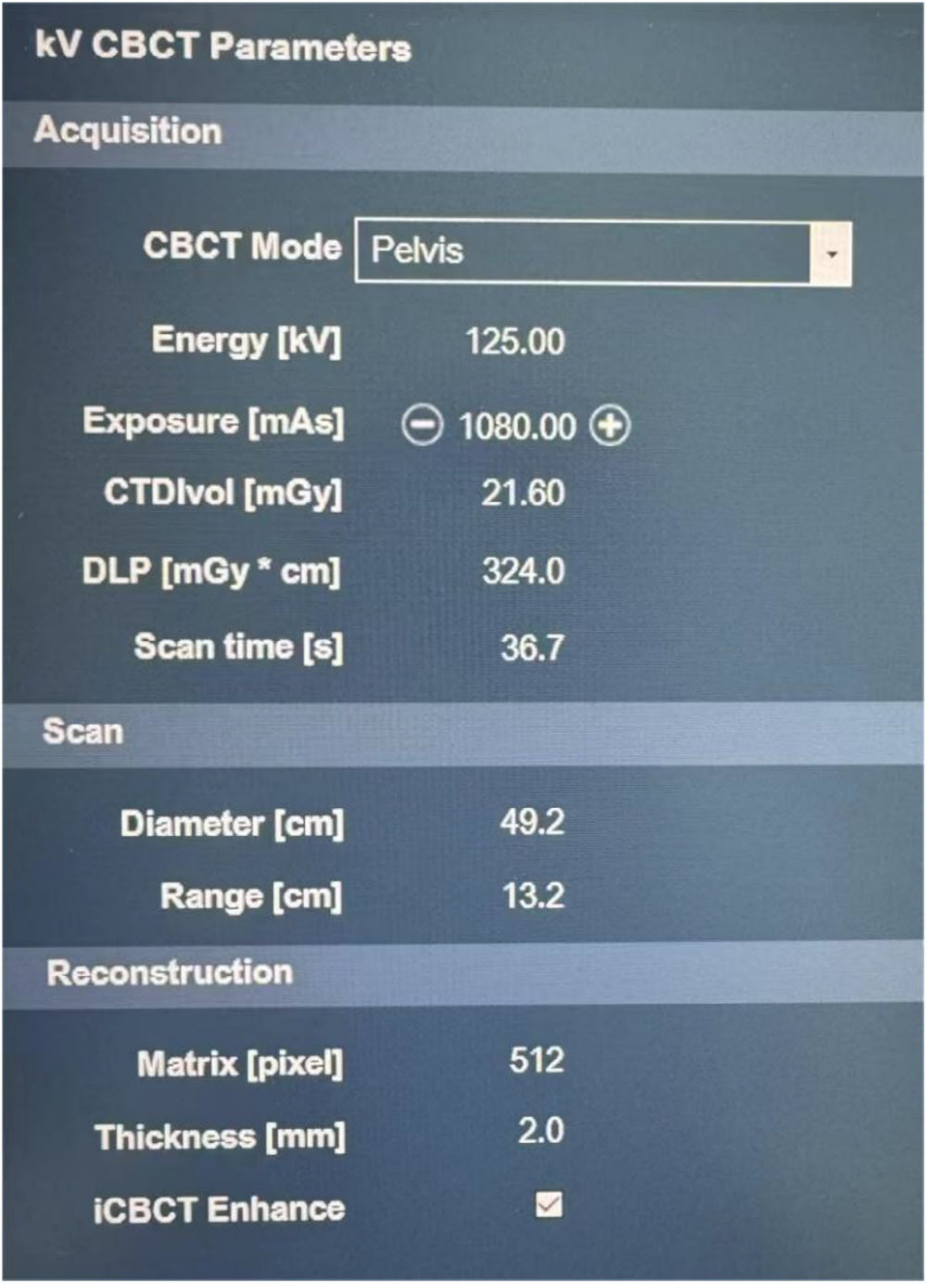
iCBCT scanning parameters.
